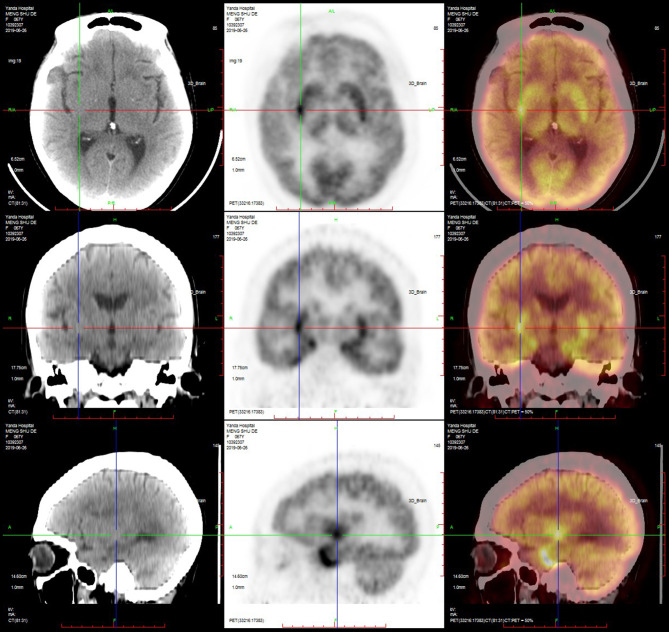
PET-CT of a typical patient. PET-CT suggested that the metabolism of the bilateral basal ganglia was increased, especially on the right side.
